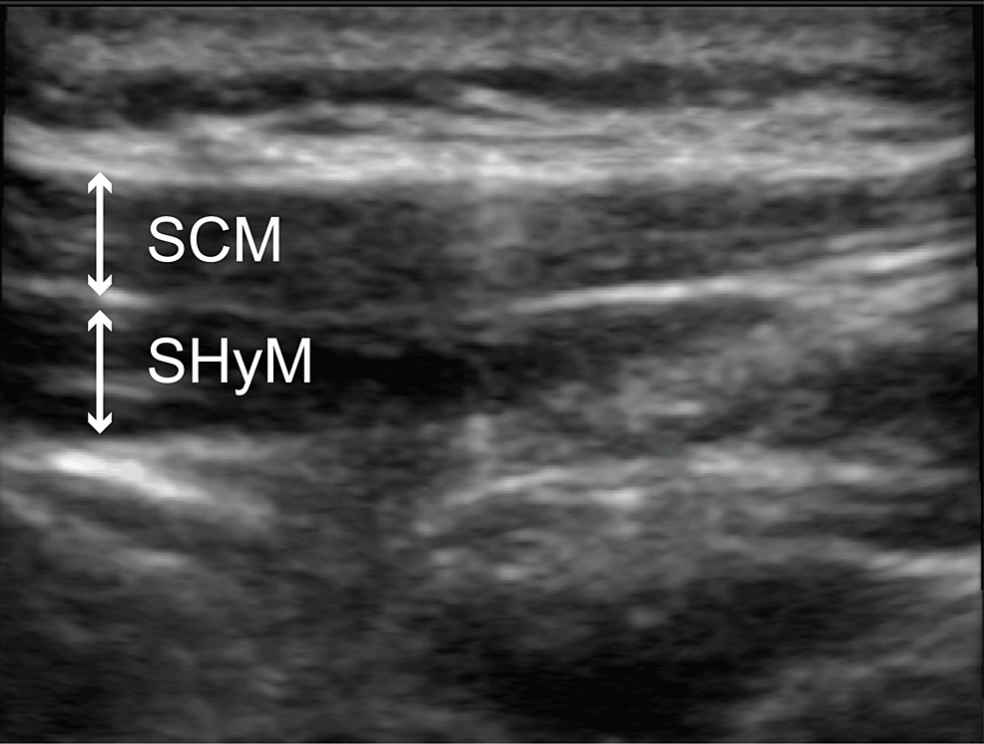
Ultrasonographic images obtained before ultrasound-guided selective glossopharyngeal nerve block in Case 1 After the sternocleidomastoid muscle is identified, a linear ultrasound probe is set parallel to the sternocleidomastoid muscle running just caudal to the mandibular ramus. SCM, sternocleidomastoid muscle; SHyM, stylohyoid muscle
